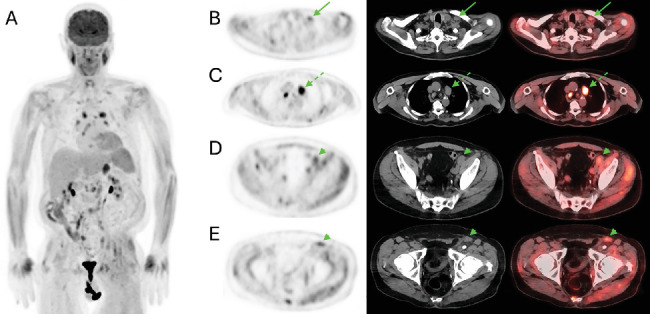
FDG PET/CT MIP (A) performed for staging of MCC approximately 1 month after the PSMA PET/CT shown in Figure 1. Increased FDG uptake was associated with the left supraclavicular lymph node (SUVmax 2.8, *arrow*, B), left external iliac lymph node (SUVmax 3.2, *arrowhead*, D), and left inguinal lymph node (SUVmax 3.3, *arrowhead*, E), with higher FDG uptake demonstrated by the subaortic lymph node (SUVmax 7.4, *dotted arrow*, C).
